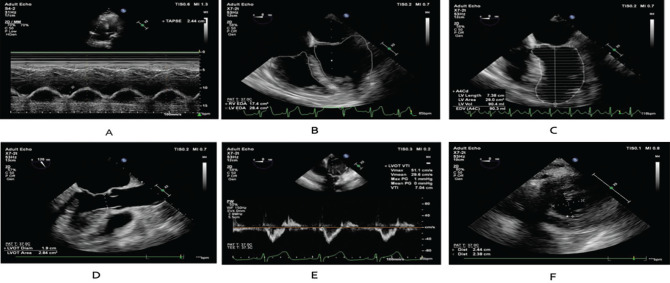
Transthorasic and transesophageal measurements A) TAPSE, B) LVEDA/RVEDA, C) LVEDV, D) LVOT diameter, E) LVOT-VTI, F) LVESEI TAPSE, tricuspid annular plane systolic excursion; LVEDA, left ventricular end diastolic area; RVEDA, right ventricular end diastolic area; LVEDV, left ventricular end diastolic volume; LVOT, left ventricular outflow tract; LVOT-VTI, left ventricular outflow tract velocity time integral; LVESEI, left ventricular end systolic eccentricity index
